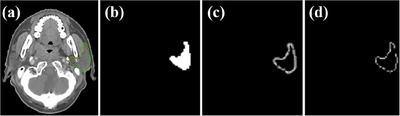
(a) The left parotid contour on CT image, (b) the predicted probability map p(i,j), (c) uncertainty map u(i,j) and (d) G(i,j): the edge area of uncertainty map detected by using second‐order Laplacian operator.
